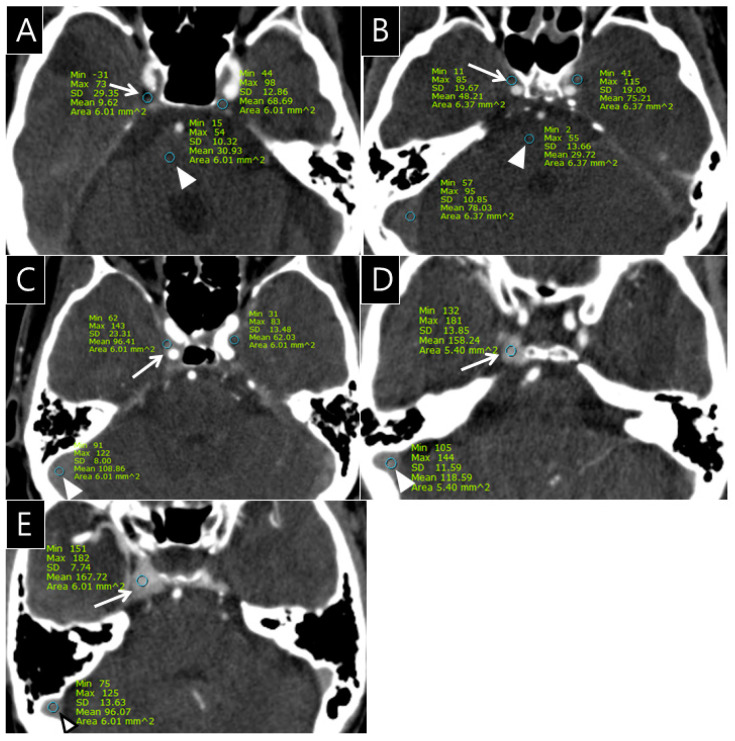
Examples of the 5-point grading scale for the cavernous sinus (CS) on computed tomography angiography (CTA); (A), Grade 0: Opacification of the right CS (arrow, CT number: 9.6 HU) is less than or equal to the density of the pons (arrowhead, CT number: 30.9 HU) on the CTA source image; (B), Grade I: Opacification of the right CS (arrow, CT number: 48.2 HU) is greater than the density of the pons (arrowhead, CT number: 29.7 HU) on the CTA source image; (C), Grade II: Opacification of the right CS (arrow, CT number: 96.4 HU) is less than or equal to that of transverse sinus (arrowhead, CT number: 108.8 HU) on the CTA source image; (D), Grade III: Opacification of the right CS (arrow, CT number: 158.2 HU) is greater than that of the transverse sinus (arrowhead, CT number: 118.6 HU) in less than or equal to one-third of the CS; (E), Grade IV: Opacification of the right CS (arrow, CT number: 167.7 HU) is seen in more than one-third of the CS (arrowhead, CT number of transverse sinus: 96.1 HU).
